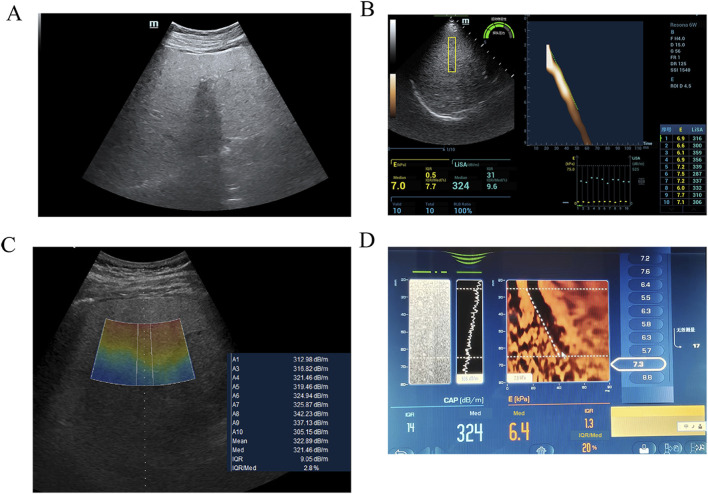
Imaging findings of severe hepatic steatosis in a 60 year-old female with hypertension. (A) B-mode ultrasound examination indicating severe hepatic steatosis. (B) UGAP measurement by GE LOGIQ E11, the value was 321 dB/m (C) LiSA measurement by Mindray Resona 6w, the value was 324 dB/m (D) CAP measurement by FibroScan, the value was 335 dB/m.
